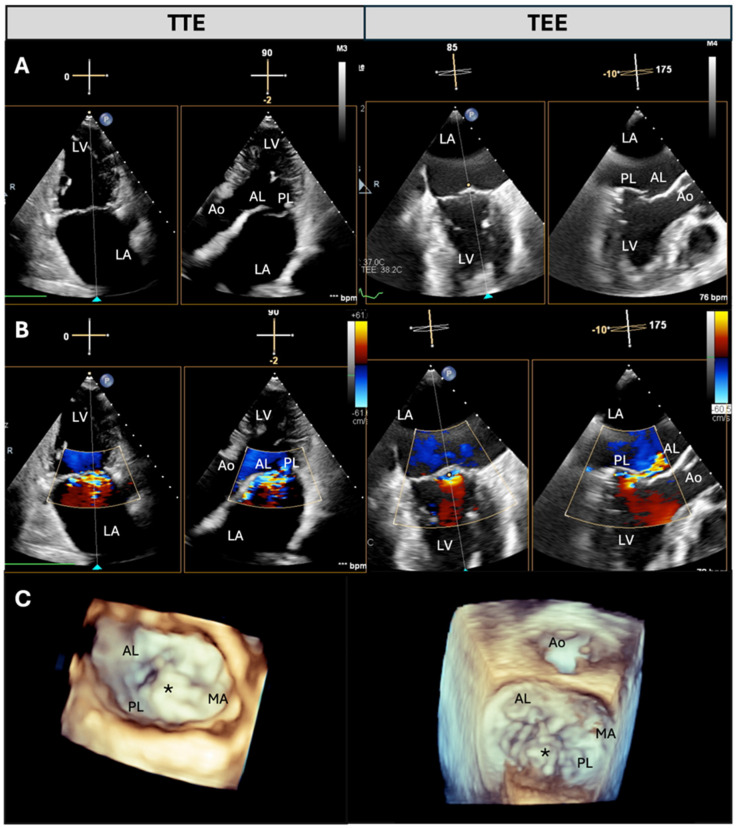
Comparison of TTE and TEE imaging in the assessment of degenerative mitral regurgitation with posterior leaflet flail. Example of degenerative mitral regurgitation with PL flail, assessed using transthoracic echocardiography (left) and transesophageal echocardiography (right). (A) B−mode images acquired using X−plane mode, showing PL flail. (B) Color Doppler images were obtained with X−plane mode, demonstrating severe mitral regurgitation with an eccentric jet. (C) 3D reconstructions of the mitral valve. The TEE 3D image clearly highlights the PL flail. * = flail segment; Ao = aorta; AL = anterior leaflet; LA = left atrium; LV = left ventricle; MA = mitral annulus; PL = posterior leaflet.
